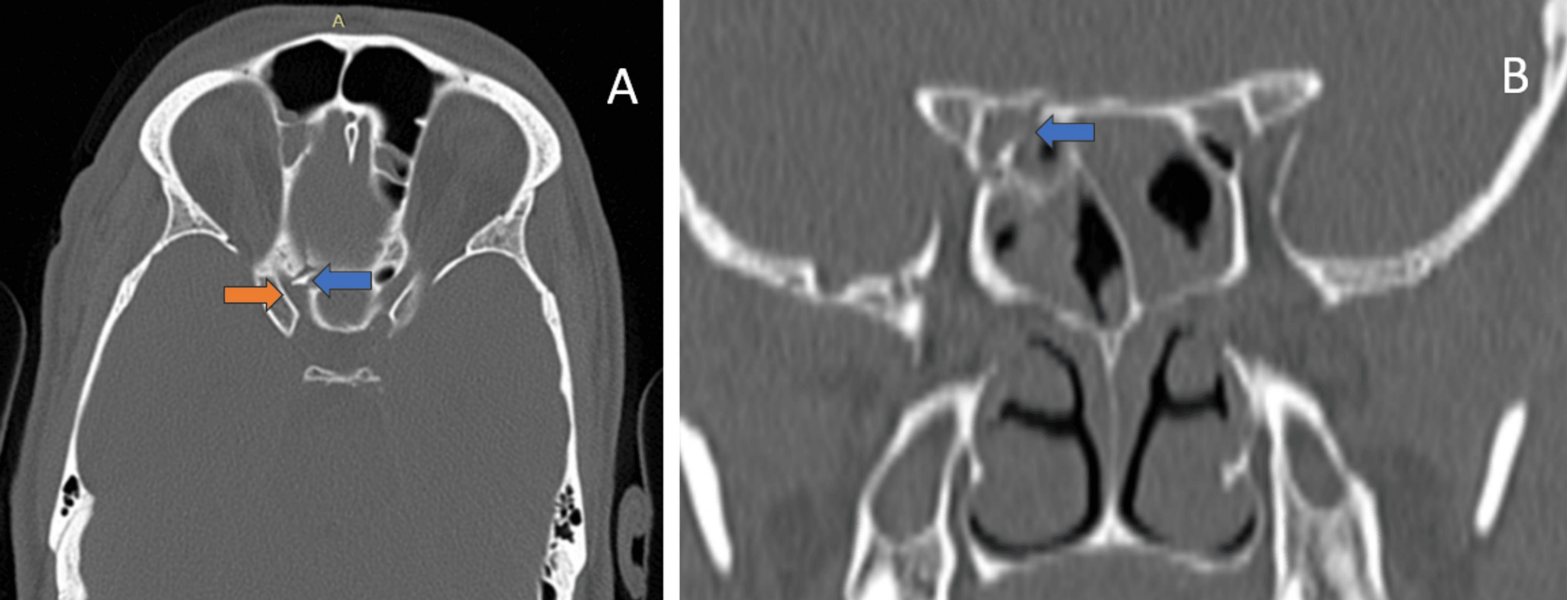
(A) Axial non-contrast computed tomography image of the orbit shows a sharp linear fracture fragment measuring 5 mm (blue arrow) impinging the right optic nerve canal (orange arrow). (B) Coronal non-contrast computed tomography image of the orbit shows a fracture of the medial wall and roof of right side optic nerve canal (blue arrow).
